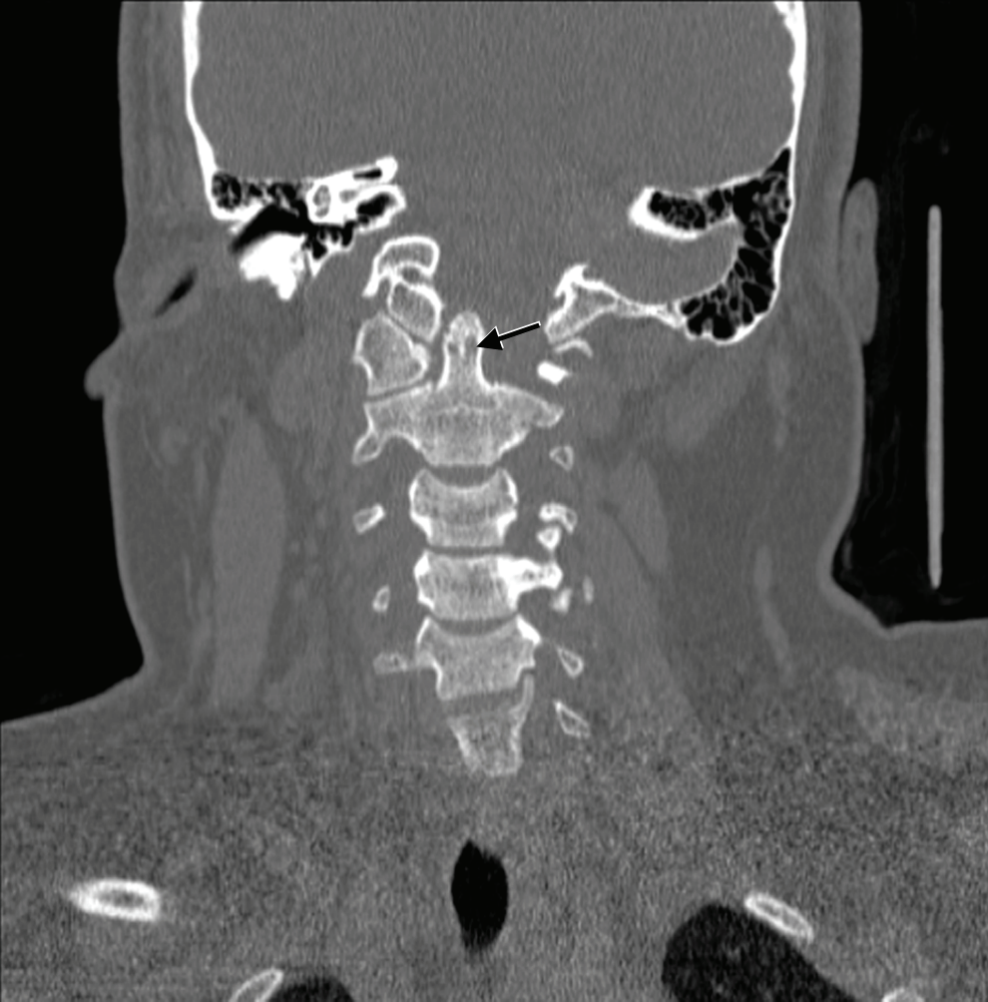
Coronal computed tomography of the cervical spine shows evidence of bony erosion at the tip of the odontoid (arrow).
